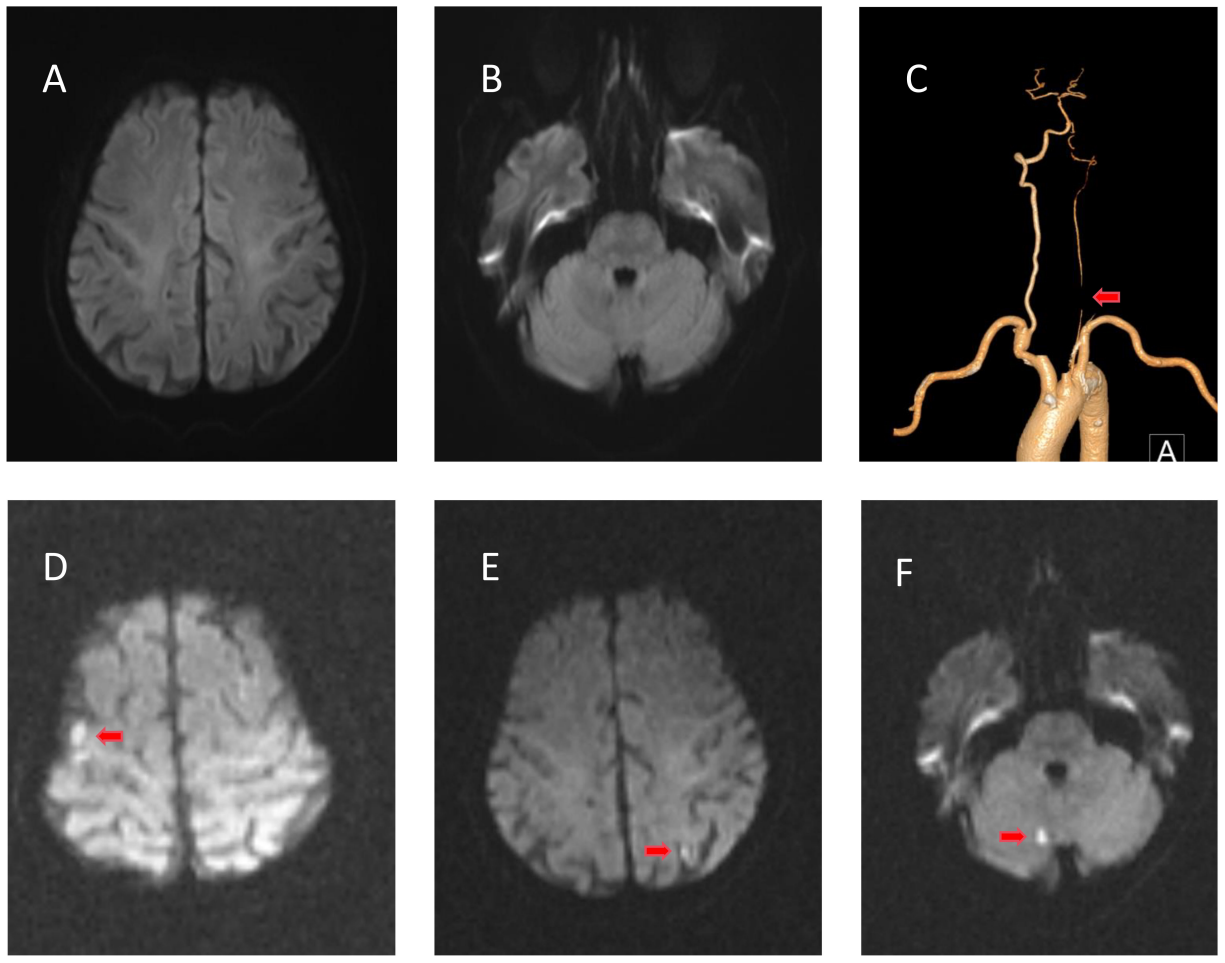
Radiological examinations of case 1 before and after intravenous immunoglobulin (IVIg). (A, B) No evidence of cerebral infarction before IVIg on diffusion-weighted imaging (DWI). (C) Computed tomography angiography showing occlusion of the intracranial segment of the left vertebral artery. (D–F) DWI of the right cerebellum, left frontotemporal cortical area, and right frontal cortical area showing restricted diffusion of the lesion after IVIg.
